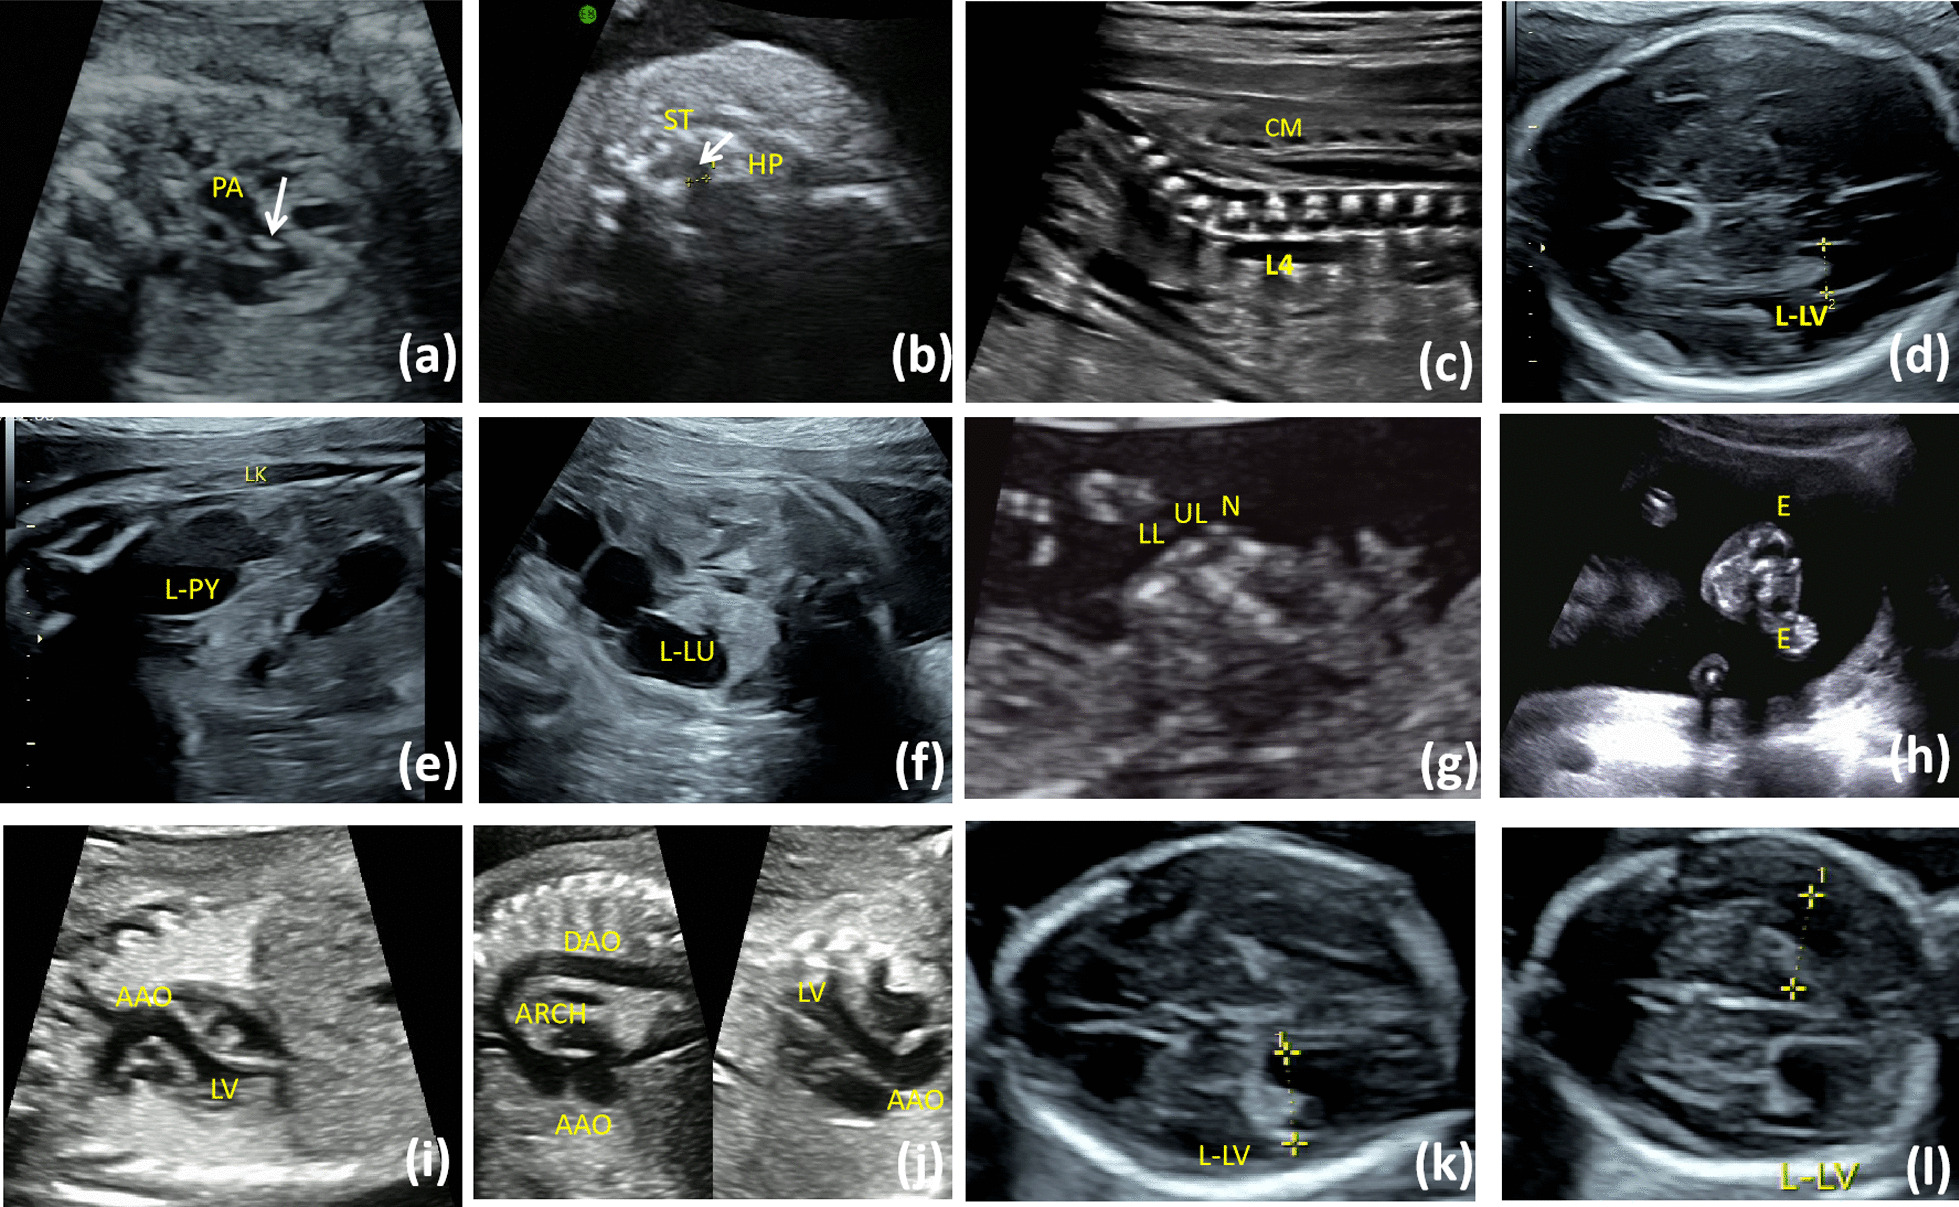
(a) The ultrasound examination of the first case with a hyperechogenic of fetal aortic valve (white arrow). b The ultrasound images of the second case in oblique view through oral fissure, showing break in echo of palatine bone horizontal plate(white arrow). c The ultrasound images of the second case showed a low-lying conus medullaris, the CM located at the L4 level. d, k, l The ultrasound view showed left mild ventriculomegaly in case 3(d), and bilateral mild ventriculomegaly in case 6 and 7(k,l). e, f The ultrasonic examination of the third case showed severe hydronephrosis of the left kidney with dilatation of the left ureter. g, h The ultrasonic examination of the fourth case showed an absent upper cranial vault and no cerebral tissue above the level of the orbits in the coronal view (g) and the coronal view (h). i, j The ultrasound images of the sixth and seventh cases showed the inner diameter of the ascending aorta was widened in the left ventricular outflow tract view and the long axis of the aortic arch view
